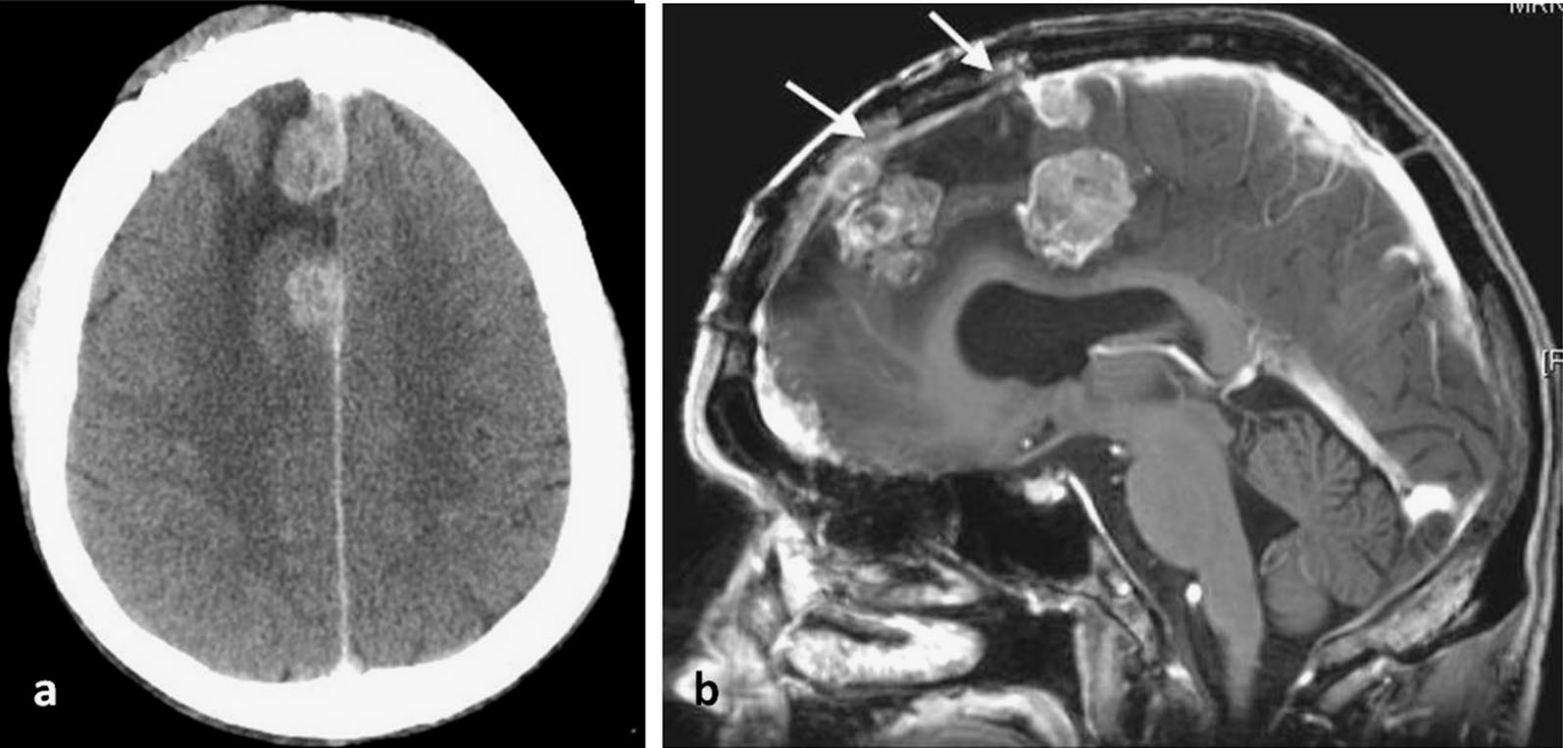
Axial CT post contrast image showing: a nodular enhancing masses and perilesional oedema in the right frontal lobe, indicative of local recurrence of the meningioma. b A sagittal T1-weighted MR image post contrast confirming the presence of three lesions and invasion of the superior sagittal sinus (*arrows*)
